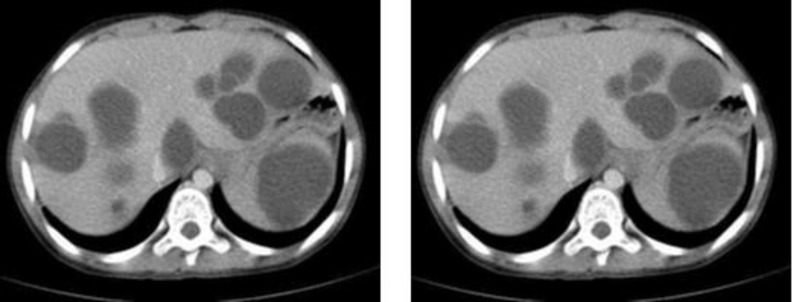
Abdominal CT scan (portal phase) with contrast reveals multiple well-defined low-attenuation mass lesions in spleen and liver without marked enhancement
